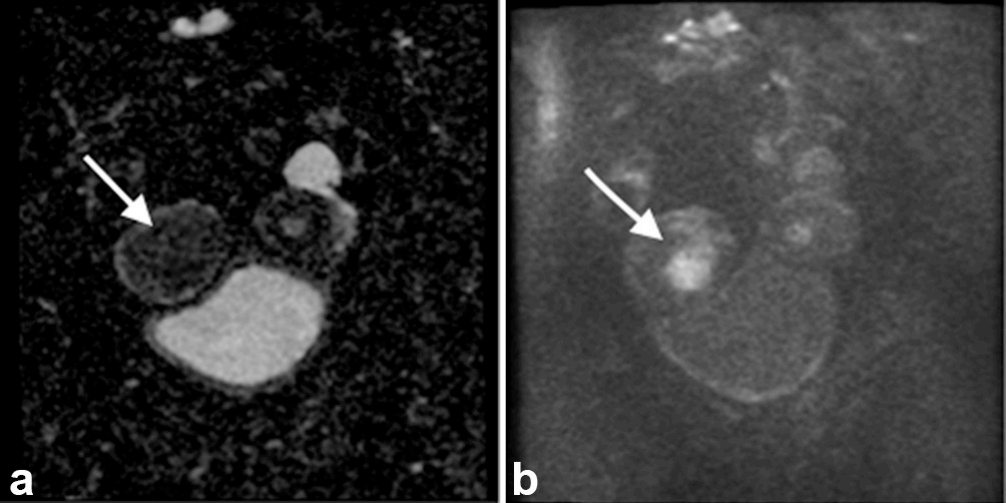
(a) MRI diffusion-weighted images. Apparent diffusion coefficient map (b) b800 reference image showing diffusion restriction pattern confirming the likely malignant nature of the right uterine tumour (see arrow).
